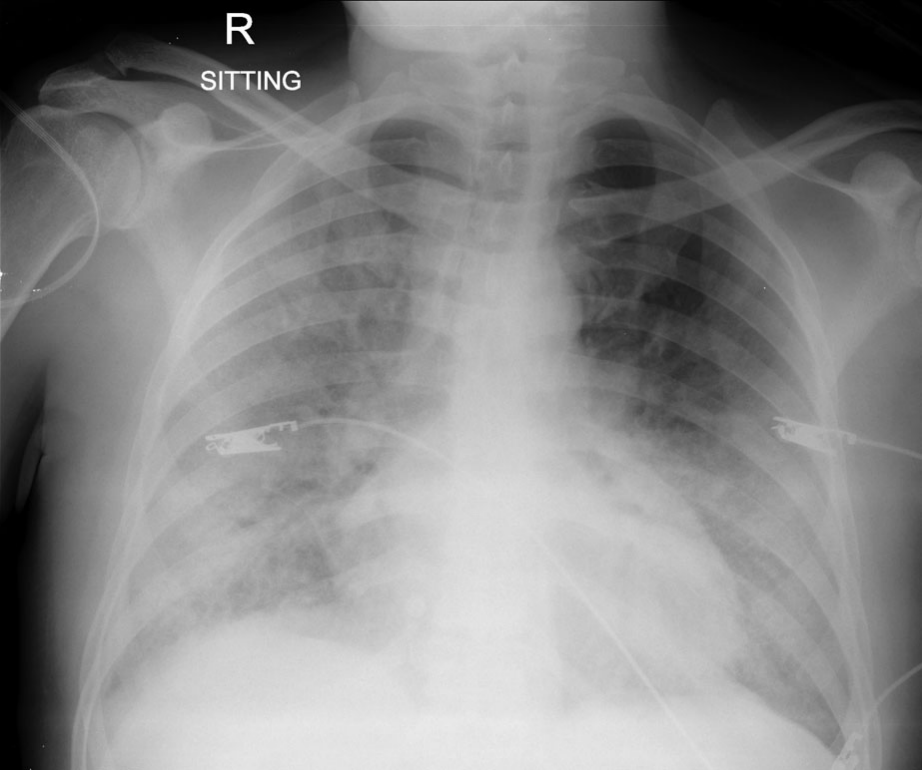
This chest radiograph showed significant worsening with consolidation involving all but the left upper zone.
